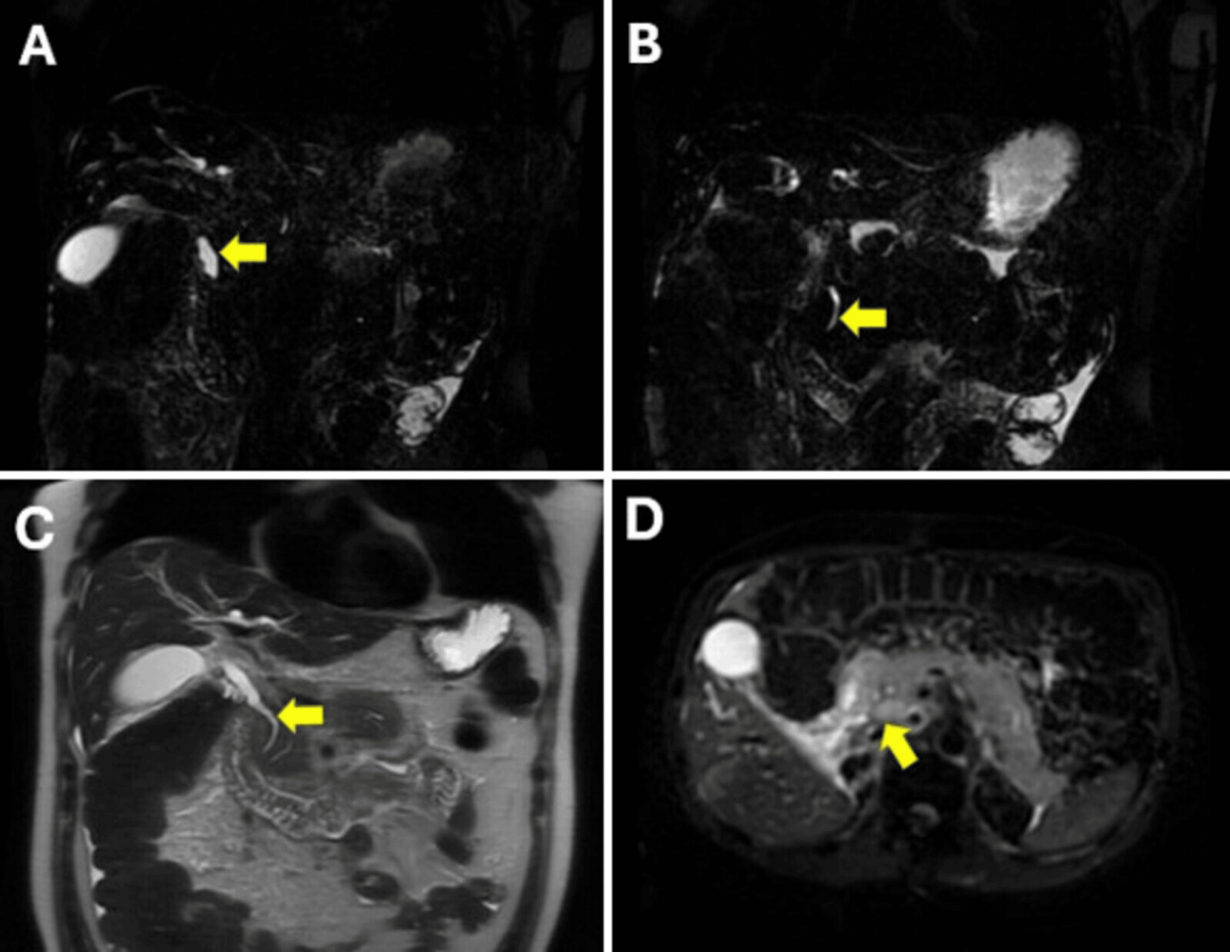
Non-contrast MRCP (A) Coronal MRCP demonstrating mid-common bile duct dilatation with (B) distal common bile duct tapering, without identifiable mass. (C) T2-weighted coronal MR image further demonstrates common bile duct dilatation with intrapancreatic narrowing, without underlying mass lesion or biliary calculus. (D) Diffusion-weighted axial (B-value=800) image demonstrating the absence of restricted diffusion at the level of the pancreatic head, consistent with the absence of visible metastatic disease at the level of the pancreas. Please note that gadolinium-enhanced MR images are not obtained due to underlying renal disease MRCP: Magnetic resonance cholangiopancreatography, MR: Magnetic resonance
